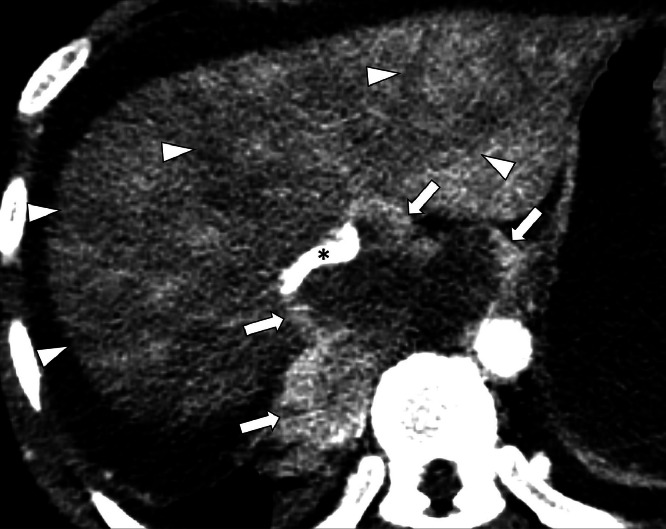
CT of mediastinal mass progression and hepatic venous congestion. Axial contrast enhanced CT of the abdomen shows a large mass in the lower chest (arrows) severely compressing and obstructing the IVC (*). The liver demonstrates heterogeneous enhancement with linear and wedge-shaped areas of decreased enhancement (arrowheads), consistent with hepatic venous congestion.
